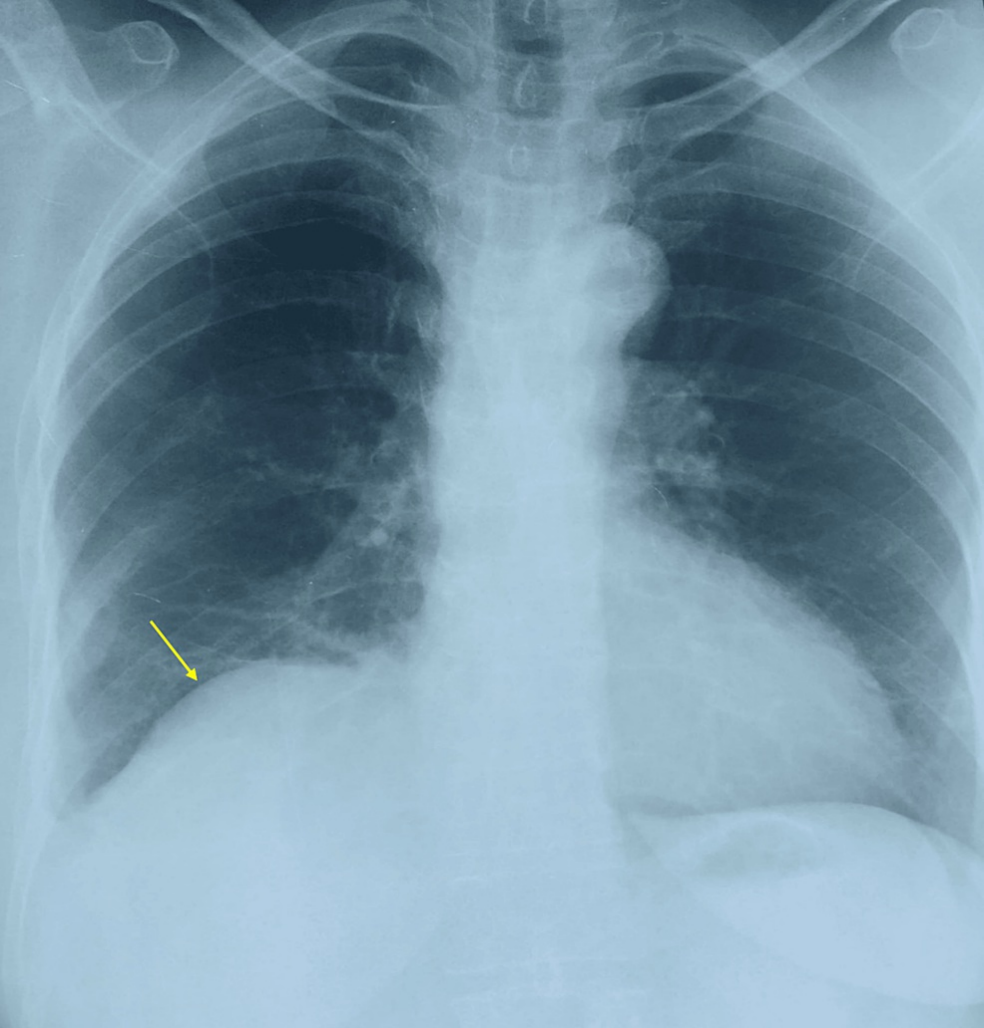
Postoperative chest X-ray showing normalization of the appearance of the diaphragmatic dome (yellow arrow) and disappearance of air-fluid images.
